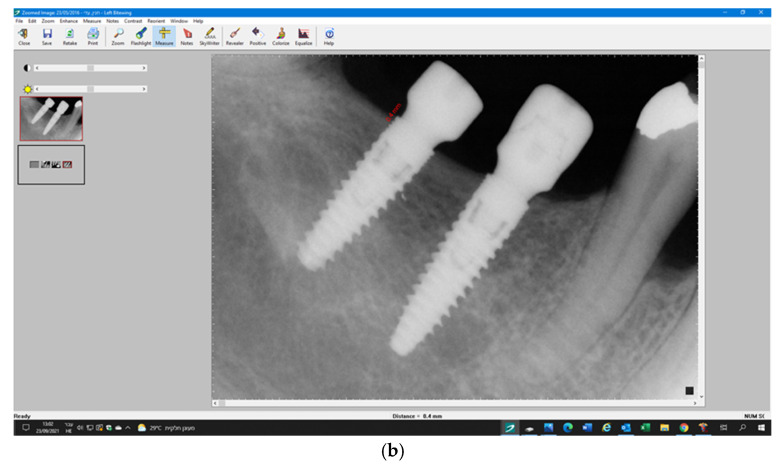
Radiographic measurements. (a). Radiographic distortion was calculated by dividing the radiographic implant length by the precise implant length using the measure (line) and calibration function. (b). Marginal bone level (MBL) was measured from the first bone-to-implant contact and implant shoulder.
